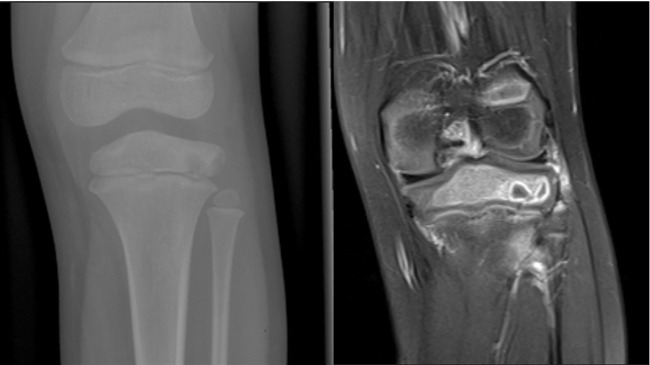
Conventional radiography and MRI of the knee in a 7-year-old child demonstrate a lytic lesion of the epiphysis due to *Staphylococcus aureus*. The MRI shows that the lesion was adjacent to the growth cartilage without crossing it. Note the presence of the “penumbra sign”; a rim of vascularized granulation tissue around the bone abscess cavity that has a higher T1 signal intensity than the cavity itself.
